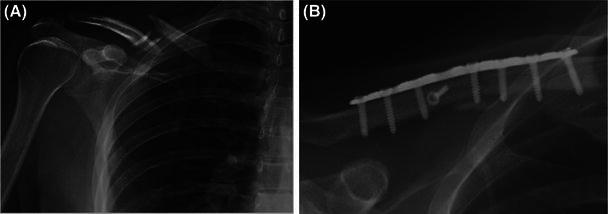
a Preoperative X-ray of 32-year-old female patient showing displaced midshaft clavicle fracture *right side*. b Immediate postoperative X-ray showing plate osteosynthesis with anatomical precontoured 3.5-mm dynamic compression plate
